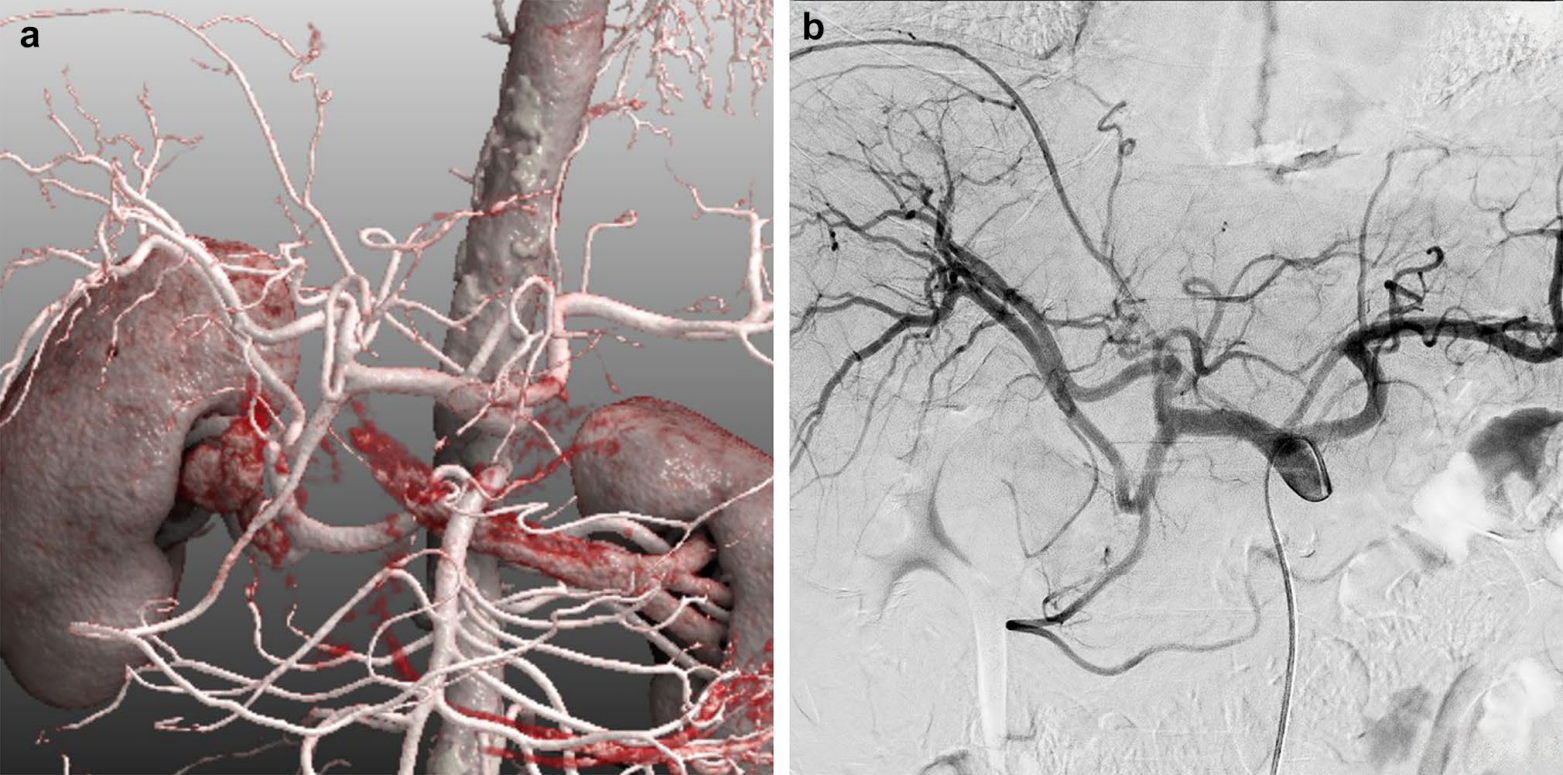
Photon-counting CT is also excellent for CT angiography (A) because of its superior spatial resolution. In this case, hepatocellular carcinoma was treated by transarterial chemoembolization, and celiac angiography was also performed during the procedure (B). CT angiography adequately delineated the hepatic artery to the periphery, which may facilitate selective catheter insertion into the hepatic artery branches
